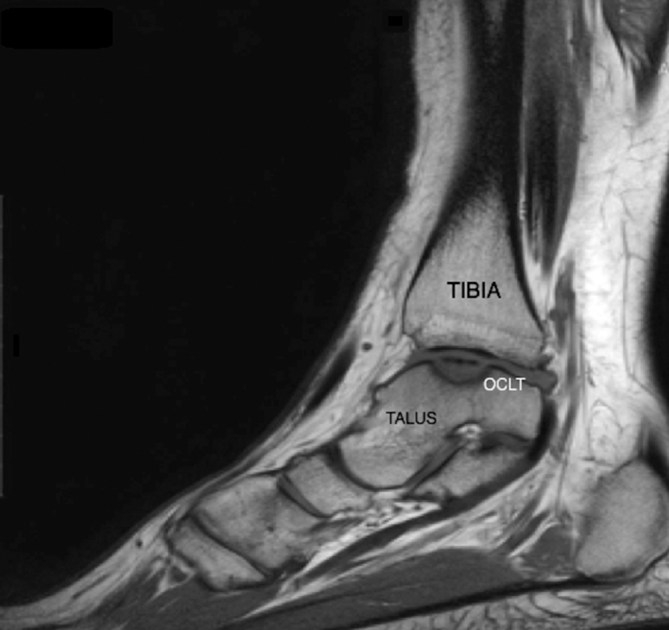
Preoperative sagittal magnetic resonance imaging scan showing a medial osteochondral lesion of the talus (OCLT). (1m, 1mm slices).
